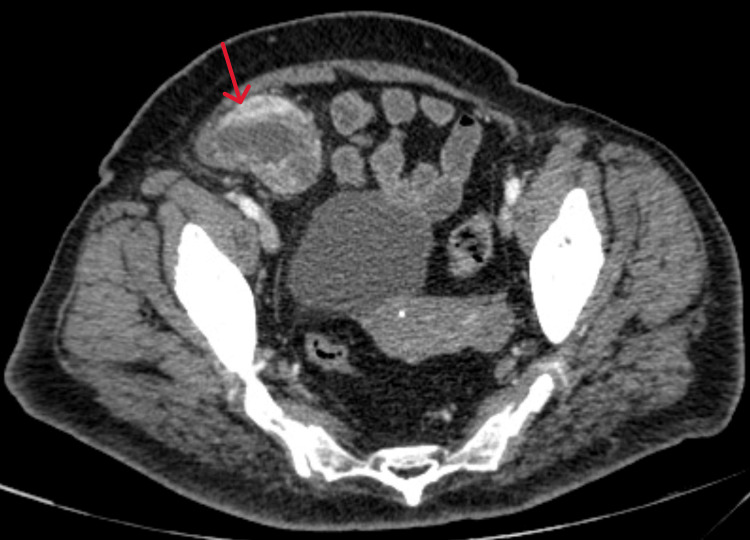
Pelvic CT with contrast showing the presence of a soft tissue mass (red arrow).
